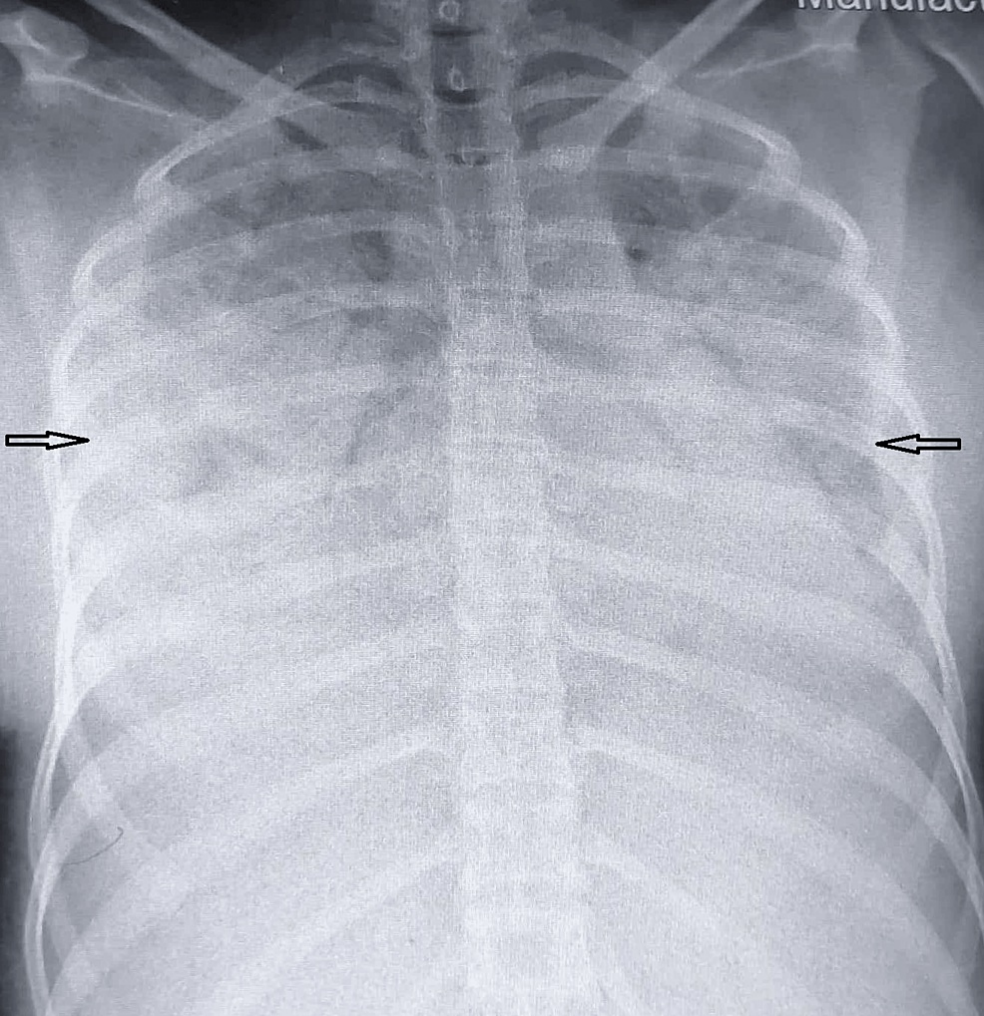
Chest radiograph on admission showing bilateral middle and lower zone opacities.
